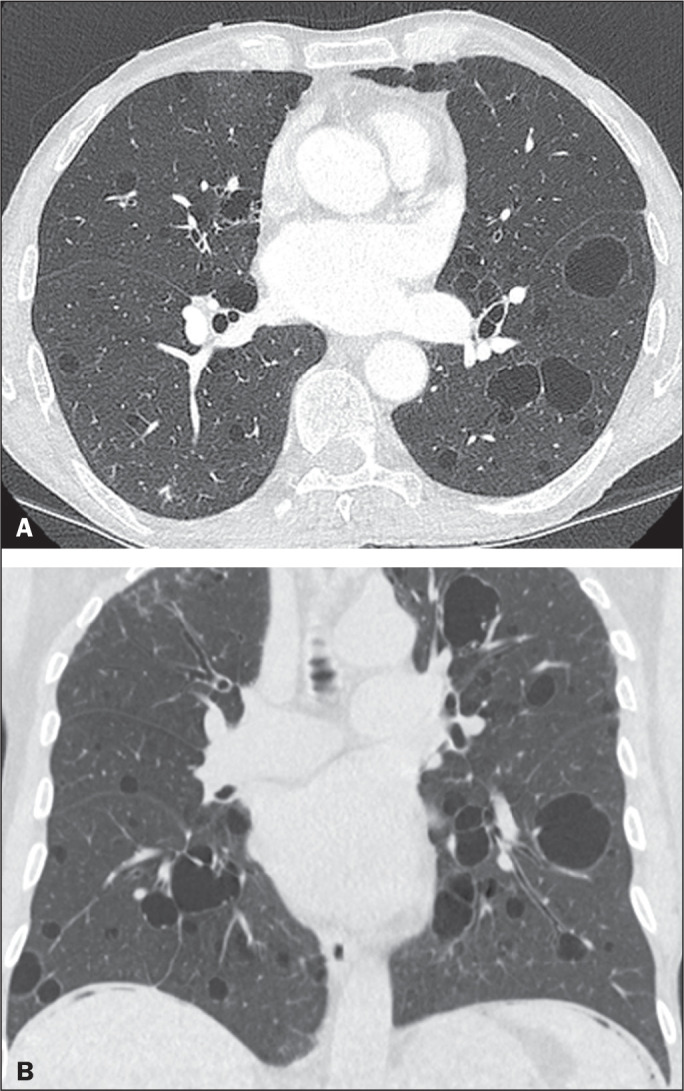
Chest CT of a 75-year-old man with NF1 who was a nonsmoker. Axial and coronal
